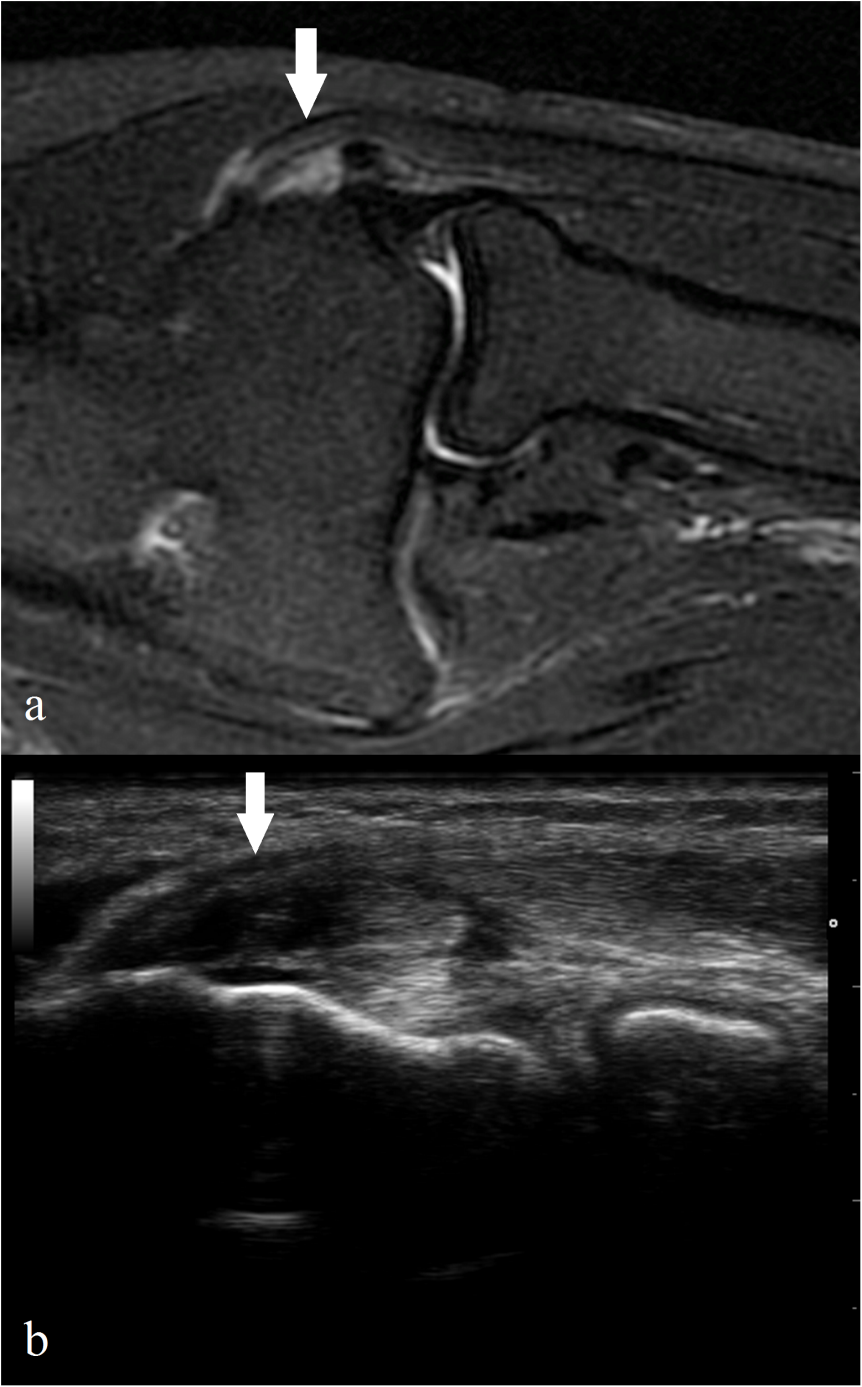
The images of high-grade CET tear (arrows) in MRI and US. Coronal fat-suppressed FSE T2-weighted image shows high-grade CET tear affecting more than 50% of the CET thickness (a). The corresponding US image of the CET tear in the same patient in longitudinal axis (b).
